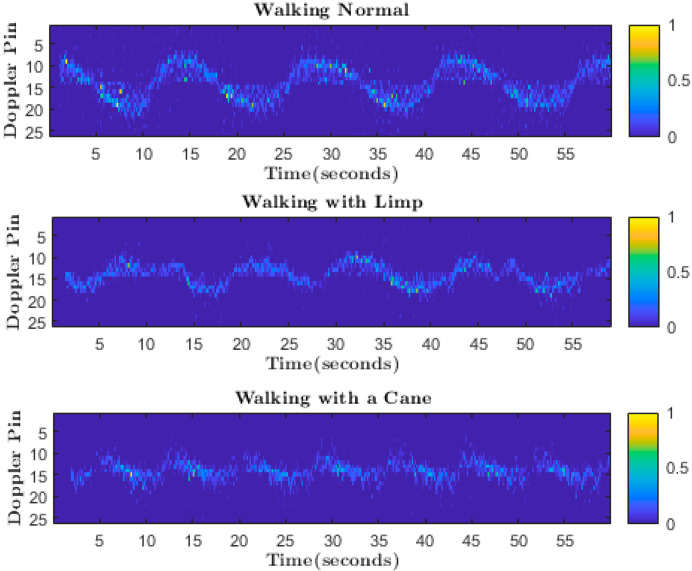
Micro-Doppler signature of different activities.
